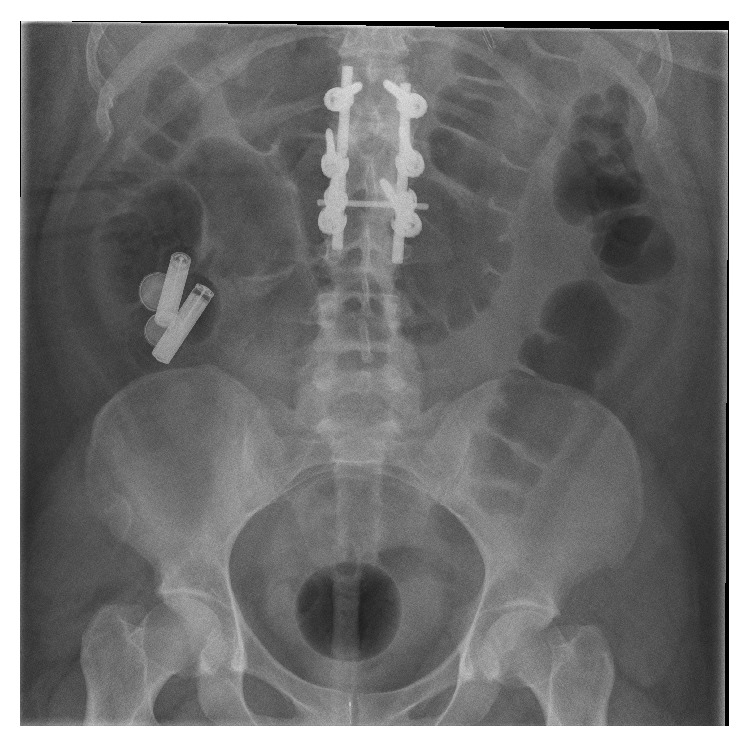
X-ray presenting a cluster of four batteries, two button batteries, and two cylindrical ones in Right Lower Quadrant (RLQ) of abdomen.
